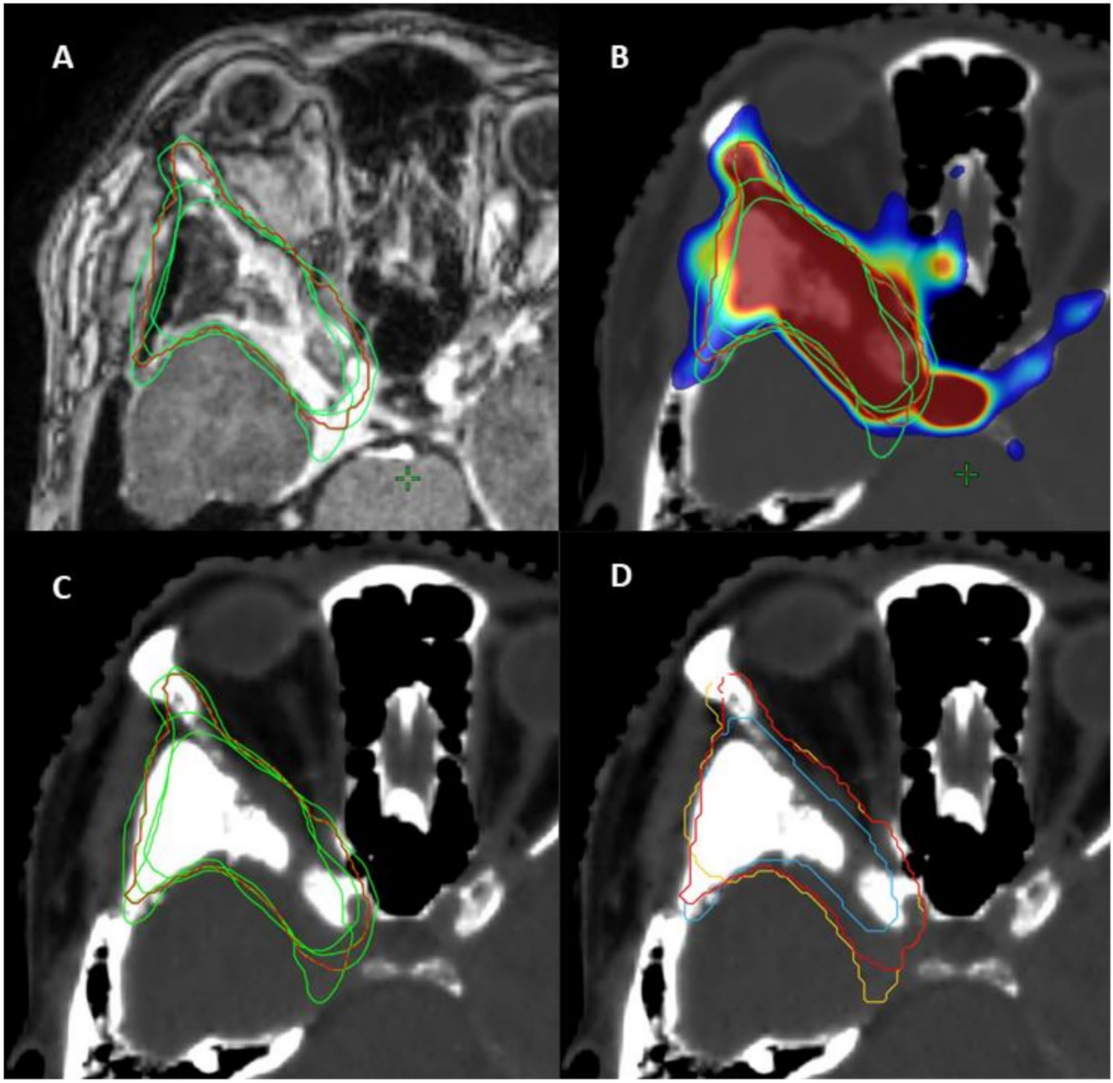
The individual GTV_ALL contours from the four radiation oncologists are shown in green, while the consensus structure (ALL_STAPLE_) is displayed in red: (A) on the MRI (T1-weighted with contrast), (B) on the SSTR PET, and (C) on the planning CT. (D) The MRI_STAPLE_ contour is depicted in blue, the PET_STAPLE_ contour in yellow, and the ALL_STAPLE_ contour in red
